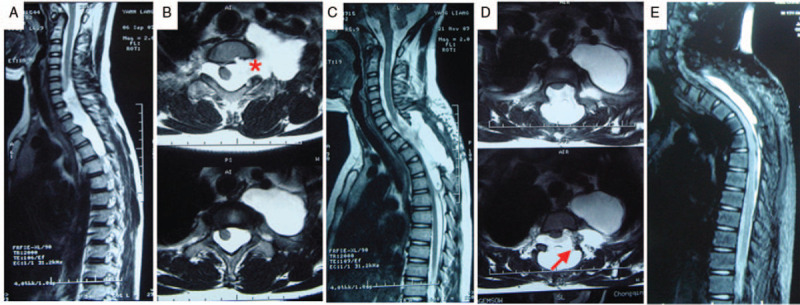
Intrathoracic meningocele in patient 4. Preoperative sagittal (A) T2-weighted MRI showing an intradural arachnoid cyst lying between C3 and T5. Axial (B) T2-weighted MRI showing a large meningocele and its connection with the arachnoid cyst at the level of T2 (red asterisk). ostoperative T2-weighted MRI (C, D) showing intrathoracic meningocele still exist with new epidural effusion. Red arrow showing the repaired site. One year after surgery, T2-weighted MRI showing shrinking of epidural effusion with a little kyphosis (E).
